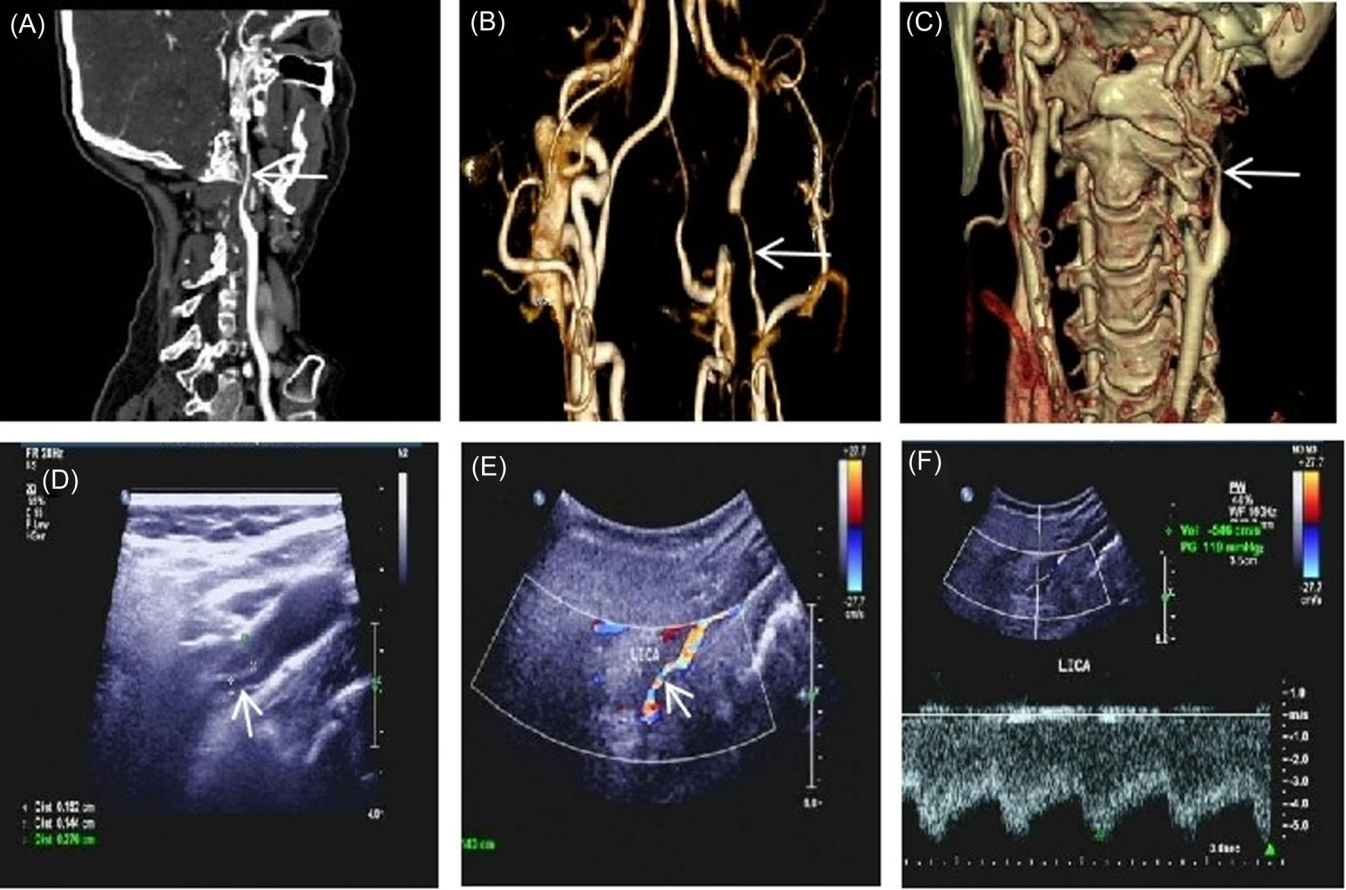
(A–C) Carotid computed tomography showing thin left internal carotid artery segment and critical stenosis of the lumen (white arrow). (D) Carotid ultrasound showing true and false double lumens in the left internal carotid artery, hypoechoic filling in the false lumen, and narrowing of the true lumen (white arrow); the residual inner diameter is about 1.4 mm and the original inner diameter was about 6.8 mm. (E) Color Doppler flow imaging examination showing no obvious blood flow signal in the false lumen, and a thin‐colored blood flow signal in the true lumen protruding outward (white arrow); the vascular diameter of the intermural hematoma is enlarged. (F) Pulsed wave measures the systolic flow rate of 546 cm/s at the stenosis of the lumen, and the distal blood flow spectrum shows low speed and low pulsation. [Color figure can be viewed at wileyonlinelibrary.com]
